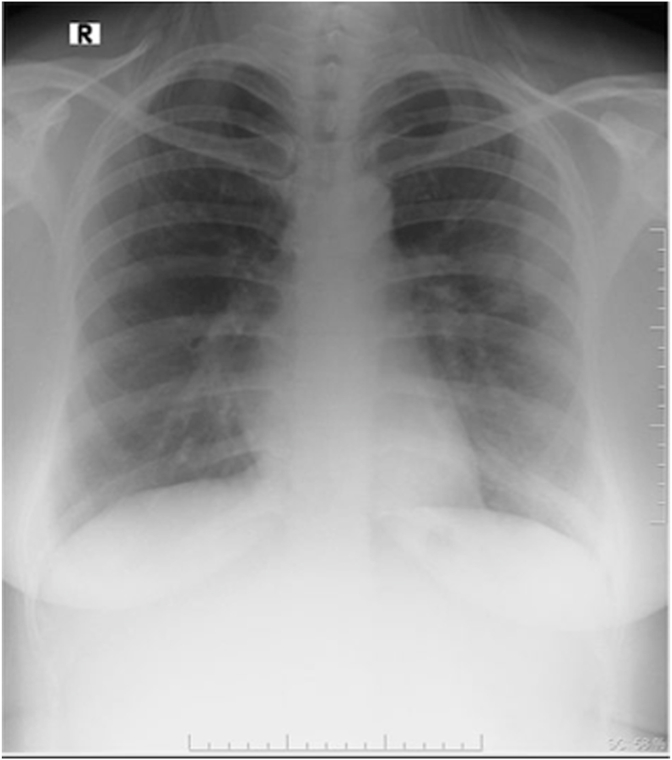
Plain chest X-ray displayed GGO in the periphery of the left lung.
